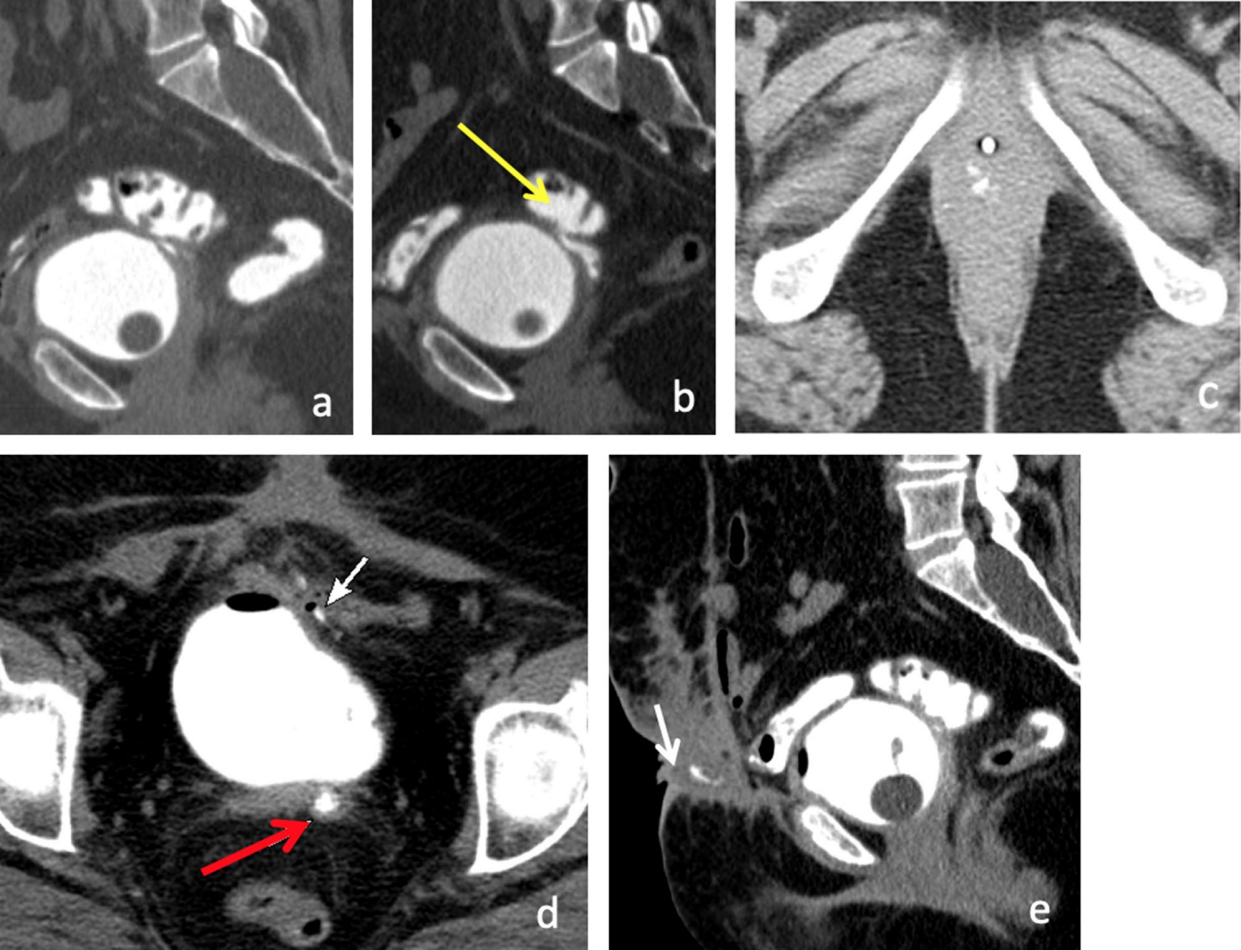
50-year-old female with history of vesicovaginal fistula after hysterectomy. CT cystogram a demonstrated opacification of the bladder and rectosigmoid colon, suggestive of a colovesical fistula located at the level of the vaginal apex. Contrast extravasation from the anterior aspect of the sigmoid colon to the vaginal apex (b, yellow arrow) is compatible with a colovaginal fistula. Additionally, a small amount of contrast is identified within the introitus of the vagina (c). Large bowel is closely adherent to the anterior pelvic wall with adjacent increased soft tissue thickening, foci of gas and contrast in the low anterior pelvic wall (d, e). These findings are suggestive of a colocutaneous fistula (white arrow). Contrast also seen in vagina (red arrow)
